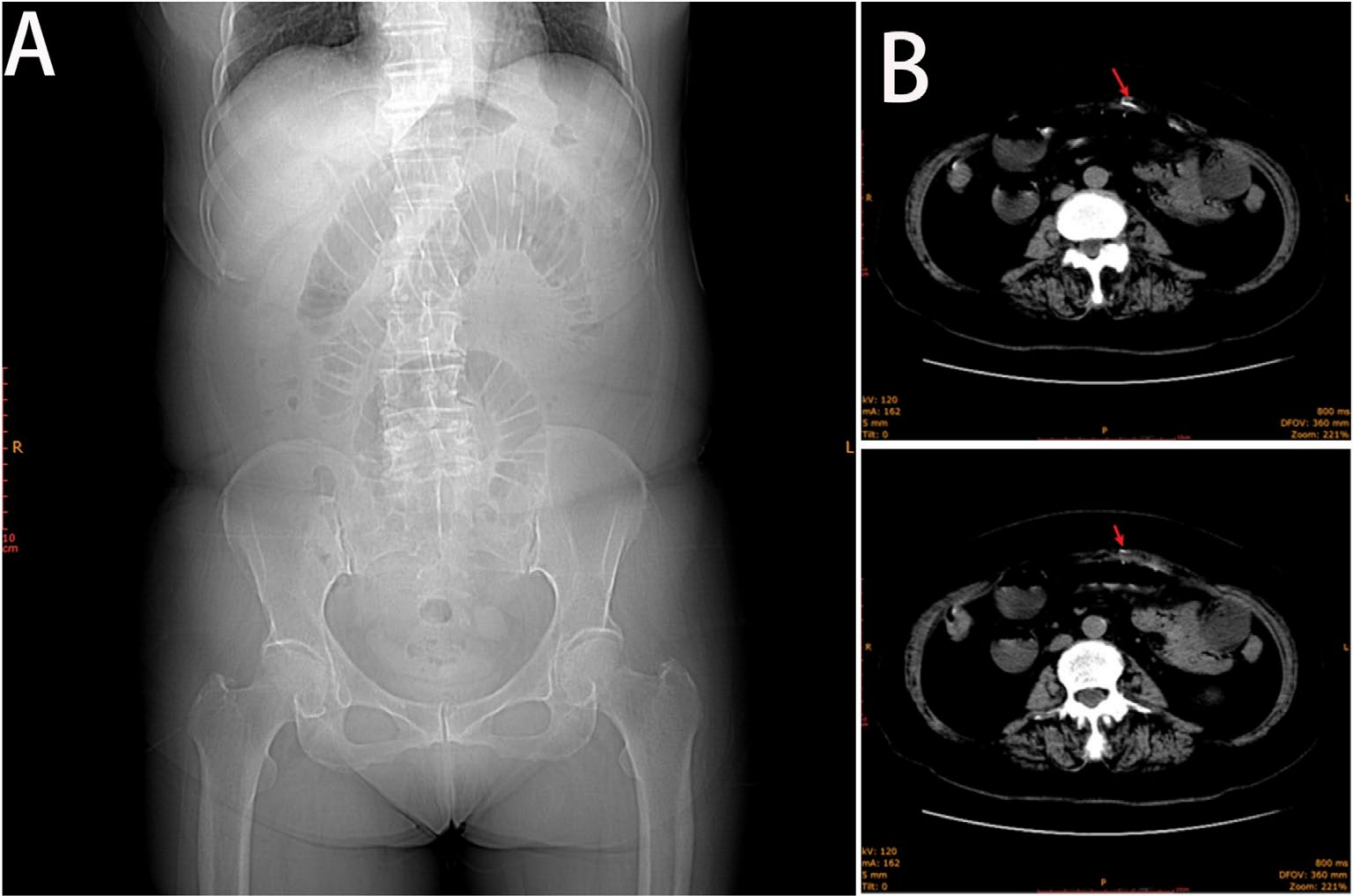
(A) CT localization image. (B) CT scan showing segmental dilation of the small intestine with intraluminal fluid and gas accumulation, formation of air‐fluid levels, and patchy high‐density shadow in the mid‐abdominal wall.
